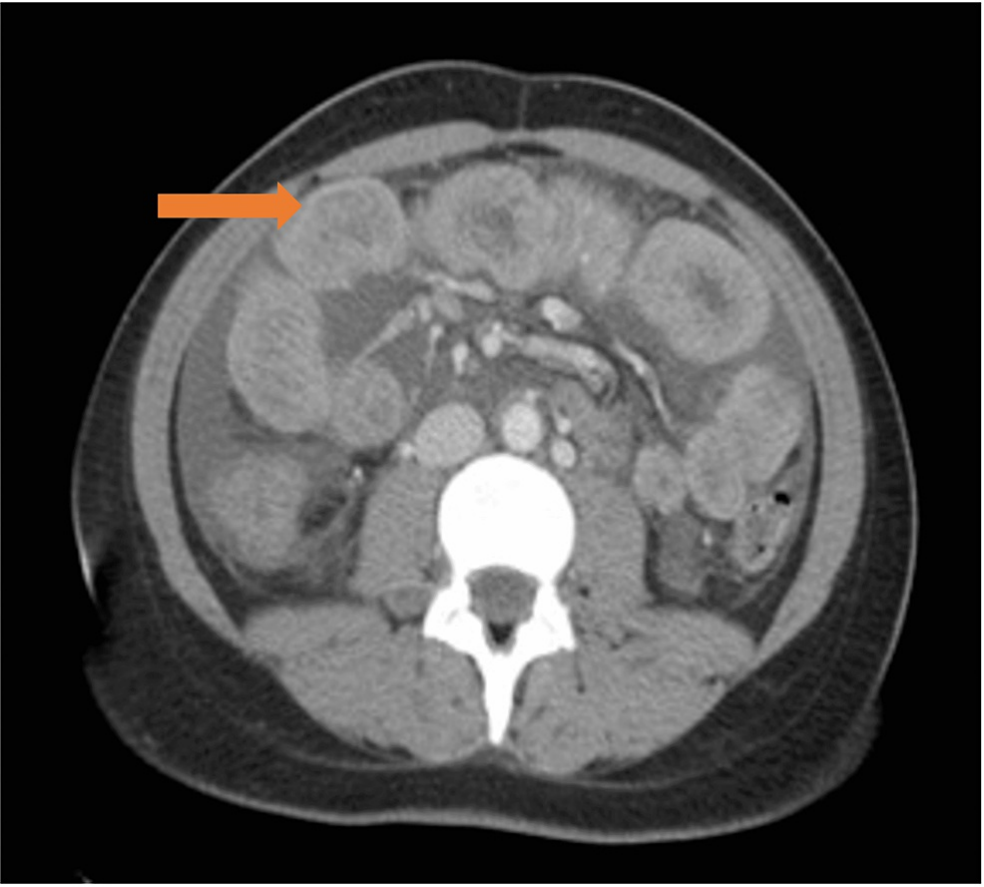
CT scan (orange arrow) highlighting parietal thickening involving duodenum and proximal jejunum of probable inflammatory nature.
